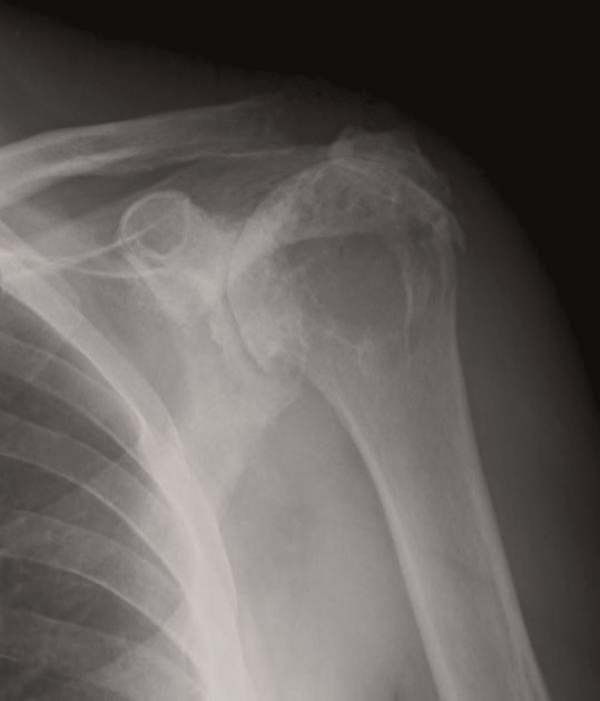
Postoperative shoulder X-ray.
